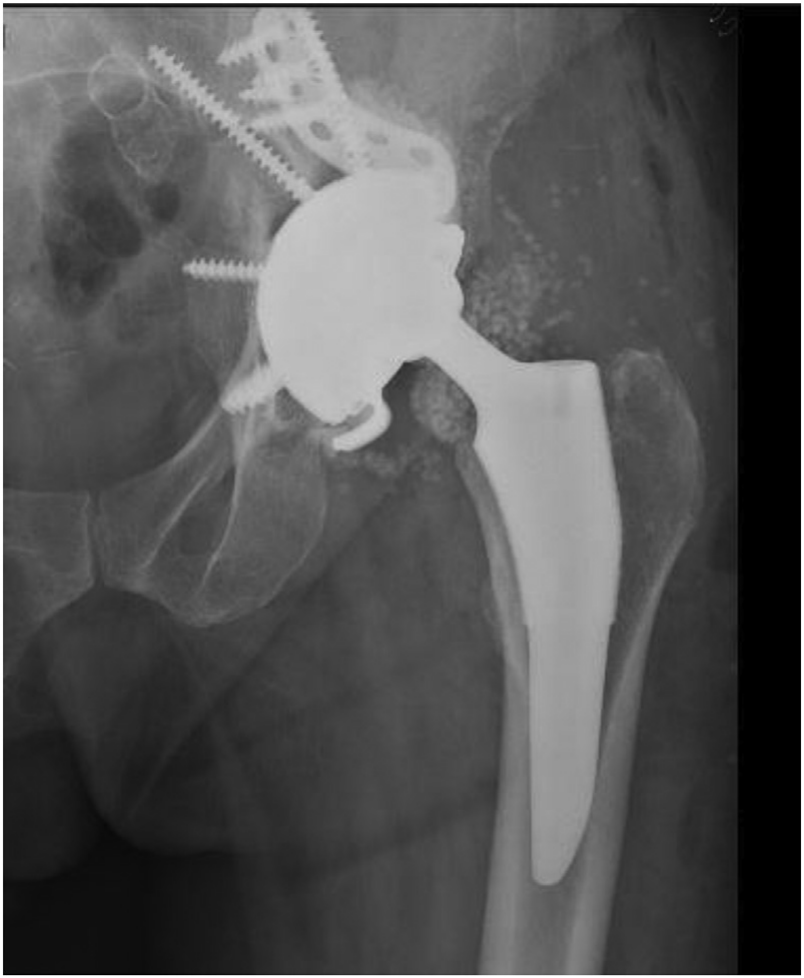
Radiograph of cup-cage construct with MDM liner.
